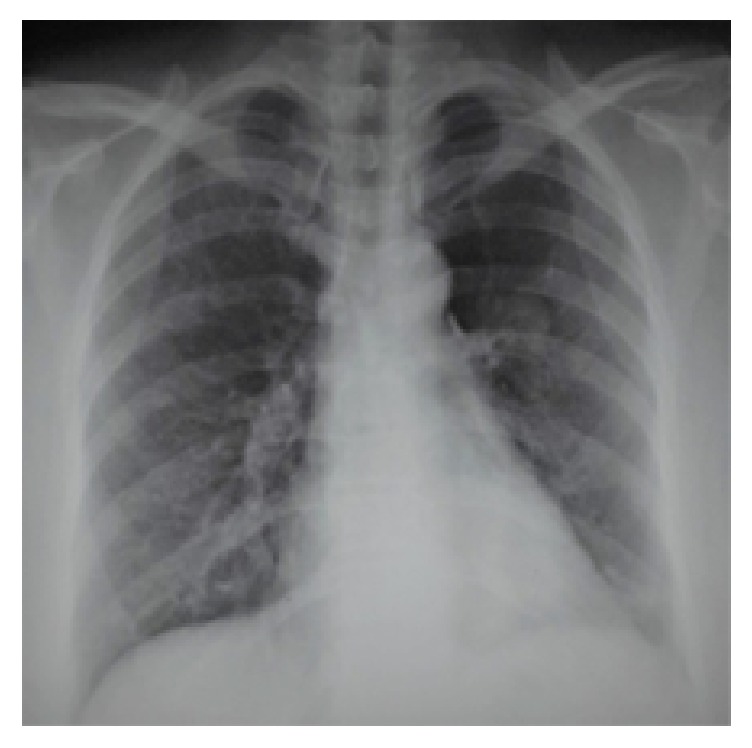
Control of chest-x-ray after cast rejection revealed complete left lung aeration.
